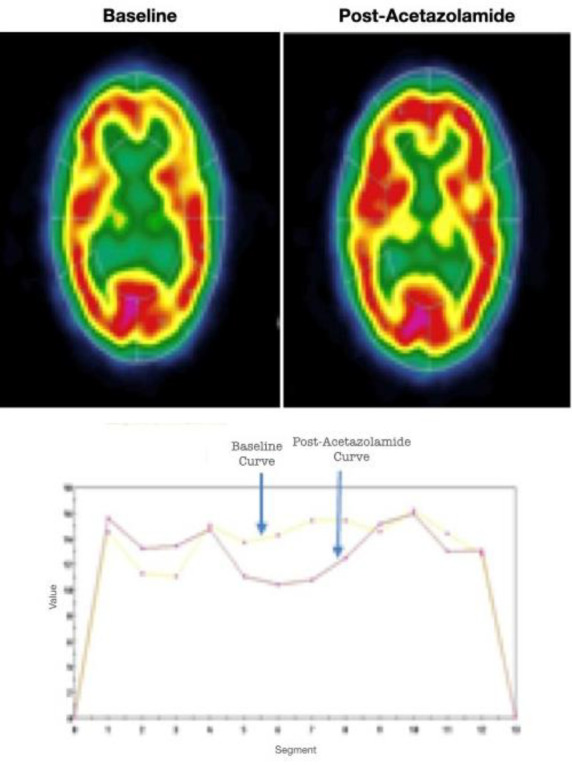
A 24-year-old female with bilateral distal ICA stenosis on MRA underwent ACZ-SPECT for CVR assessment. Post-ACZ images showed improved perfusion in multiple regions, consistent with a Type I response indicating preserved cerebrovascular reserve
